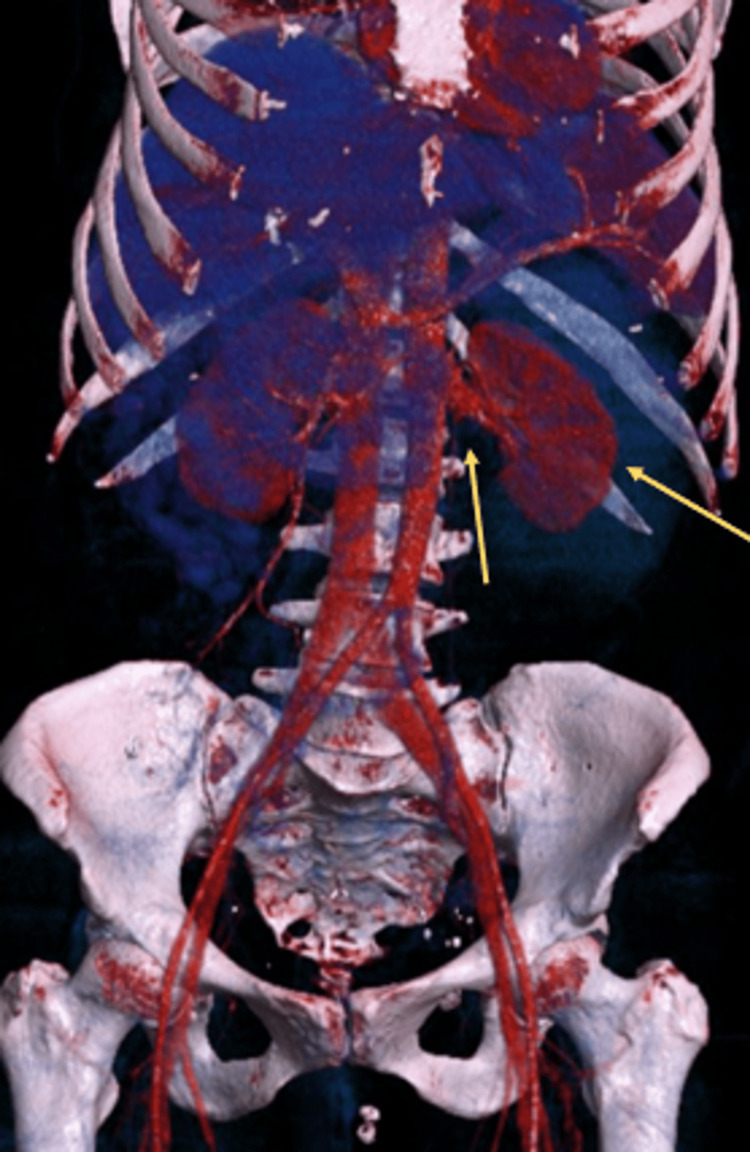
Contrast-enhanced abdominal CT (3D reconstruction) No vascular malformations or specific bleeding source are identified (yellow arrow).
